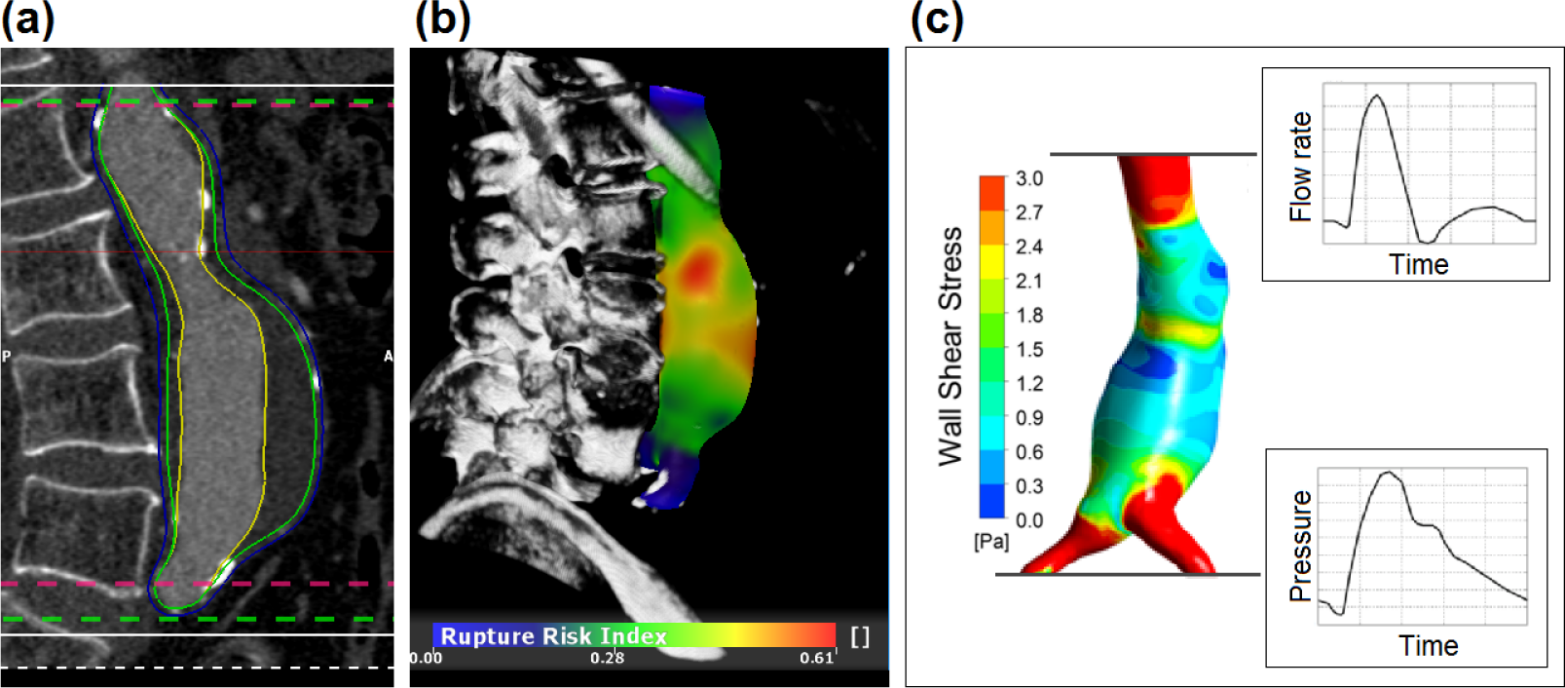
Analysis method performed for each patient at each time point. (a) Lateral Computed Tomography-Angiography (CT-A) slice with segmented Abdominal Aortic Aneurysm (AAA). Yellow, blue and green curves denote the luminal surface, exterior surface and wall-thrombus interface, respectively. (b) Rupture risk index plot derived from the structural biomechanics-based analysis at Mean Arterial Pressure (MAP). (c) Wall Shear Stress distribution at t = 0.25 s of the cardiac cycle derived from a Computational Fluid Dynamics (CFD) computation. At the inlet and the outlets, the indicated volume flow rate and pressure versus time responses were prescribed [36,37].
